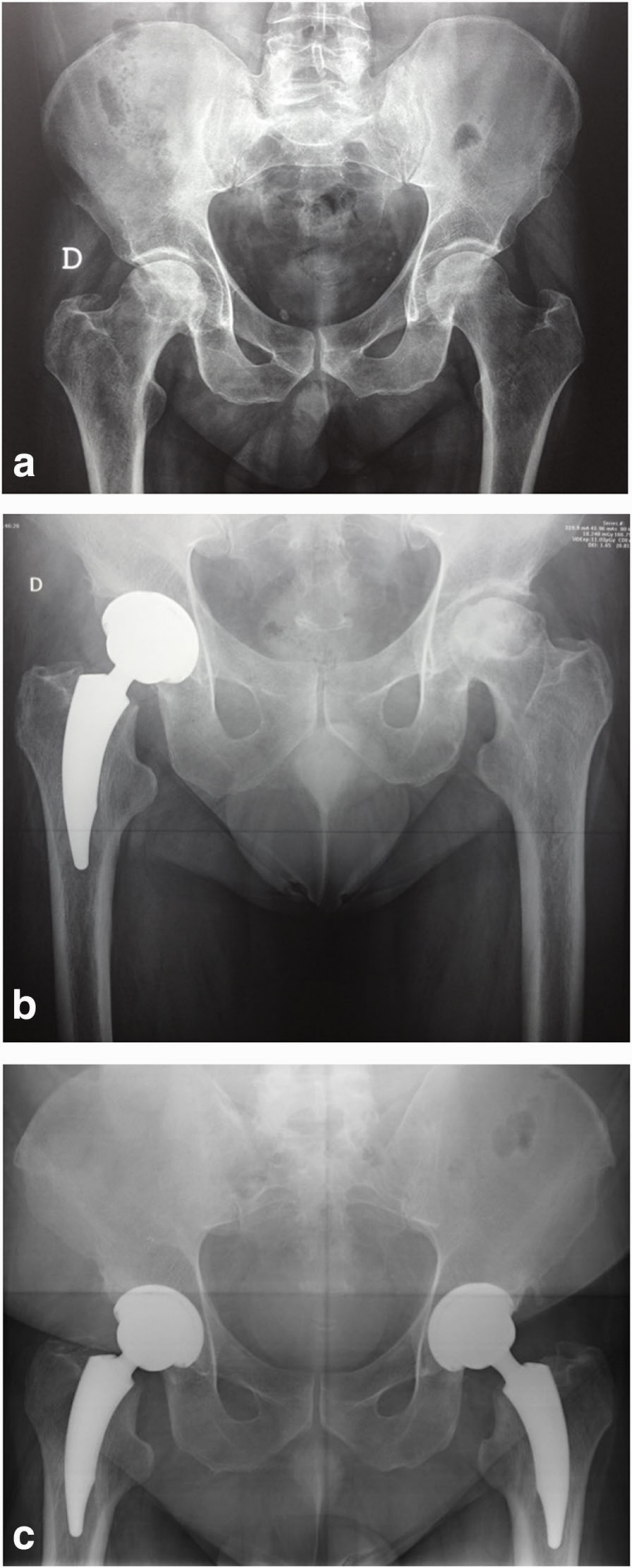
a-c Radiographs show the case of a 48-year-old male patient who had osteonecrosis of both femoral heads. a AP view of the pelvis before surgery shows Steinberg Stage IV osteonecrosis of the right femoral head. b AP view of the pelvis taken 1 years postoperatively reveals that acetabular and femoral components are well fixed in a satisfactory position; the left hip shows Steinberg Stage V osteonecrosis of the left femoral head. c At 7-year follow-up AP view Grade 1 calcar resorption is evident in both hips, without signs of stem loosening and osteolysis
